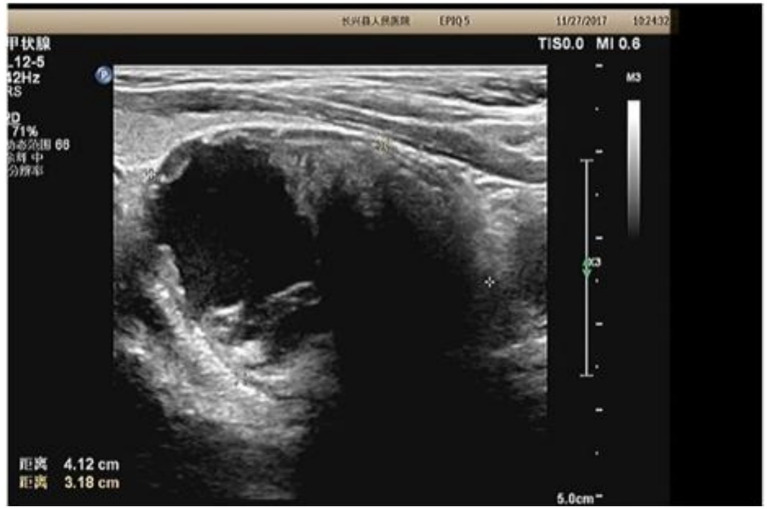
Preoperative ultrasound of the thyroid gland thyroid (ultrasound)|parathyroid findings were: multiple cystic solid nodules of the thyroid gland (TI-RADS category 3), cystic solid mass posterior to the inferior pole of the thyroid gland in the right lobe with calcification (cystic solid echogenicity of 41 × 32 mm), and parathyroid adenoma with hemorrhagic cystic degeneration. Parathyroid (ultrasound) findings were: cystic solid mass with calcification posterior to the inferior pole of the thyroid gland in the right lobe, and parathyroid adenoma with hemorrhagic cystic degeneration.
